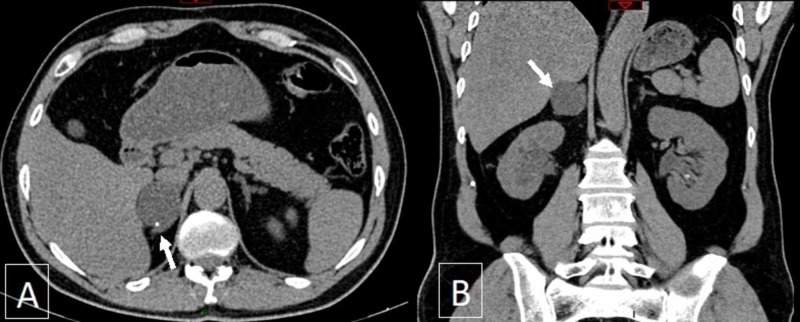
Plain CT scan of the abdomen and pelvis showing a well-defined and rounded cystic lesion arising from the median limb of the right adrenal gland (arrow), measuring 4.0 x 3.7 x 3.4 cm with a fluid density of 12 HU A small calcification is seen at its rim (arrowhead). A: axial view. B: coronal view. CT, computed tomography; HU, Hounsfield unit
